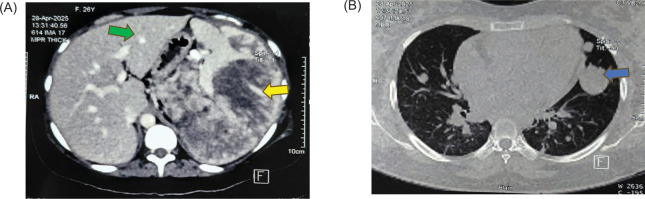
CT scan of chest and abdomen after delivery in April 2025 showed large left recurrent abdominal mass (Yellow Arrow) with extensive liver (Green Arrow) and lung metastasis (Blue Arrow).
